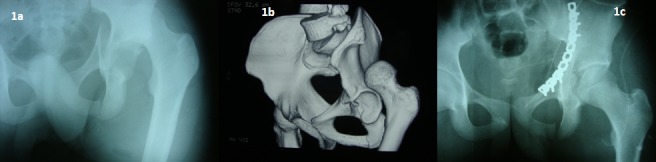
a) Pre operative AP radiograph; Preoperative radiograph clearly showing the anterior dislocation of the hip, disruption of anterior column of the acetabulum and the ischial ramus fracture; b) CT image, 3D CT image depicting the position of the femoral head. Note that there is no bony obstacle to reduction; c) Post operative radiograph, Post operative anteroposterior radiograph showing concentric hip reduction and accurate reduction and fixation of the fracture with a 10 hole 3.5 mm reconstruction plate
